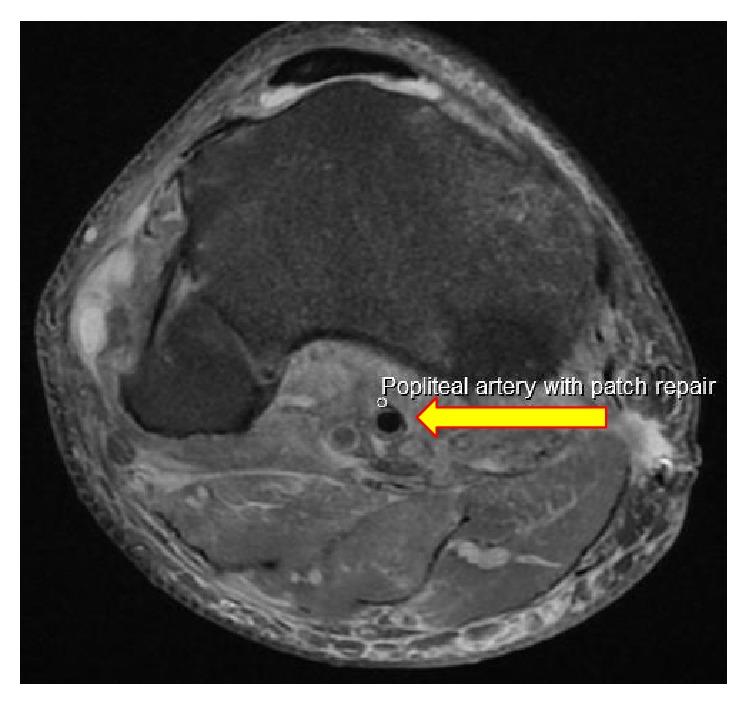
Postoperative MRI assessing multiligamentous disruption showing popliteal vessel patency and patch repair.
